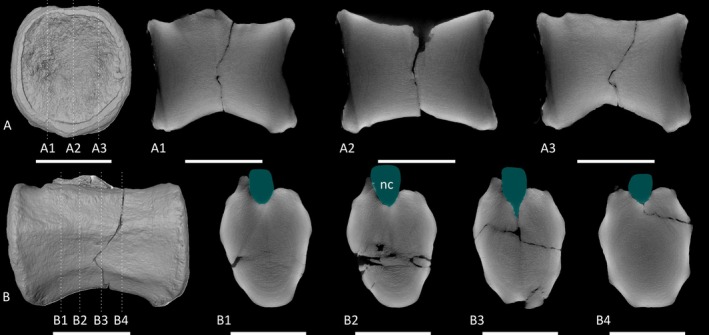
CT scan of ‘*Cetiosaurus glymptonensis*’ middle caudal vertebra OUMNH PAL‐J.13751. (A–B) 3D rendering (A) anterior view, (B) left lateral view. (A1–A3) CT scan slices in parasagittal cross‐section. (B1–B4) CT scan slices in transverse cross‐section. nc, neural canal. Key: blue highlight. Scale bars = 100 mm.
